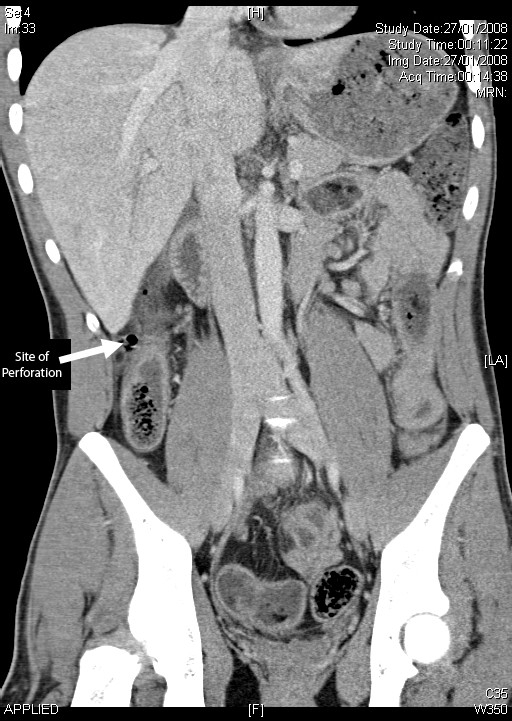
Computed tomography image of ascending colon perforation – coronal view.
